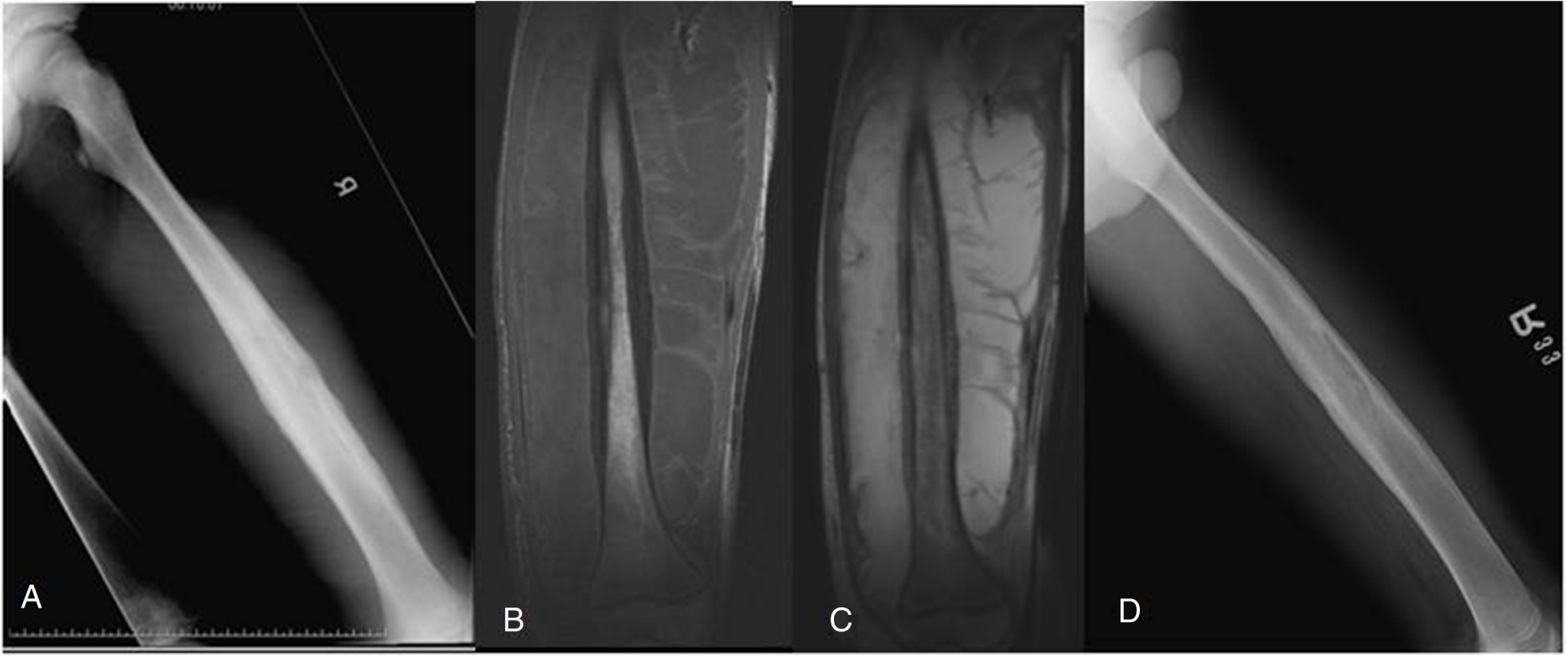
A 13-year-old boy who complained of right thigh pain for one month. (A) Plain film of the femur showing a sclerotic border with septated periosteal reaction. (B, C) T1 and T2-weighted magnetic resonance imaging (MRI) showing septated periosteal reaction with extensive soft tissue swelling. (D) Plain film radiograph taken one year after debridement surgery.
